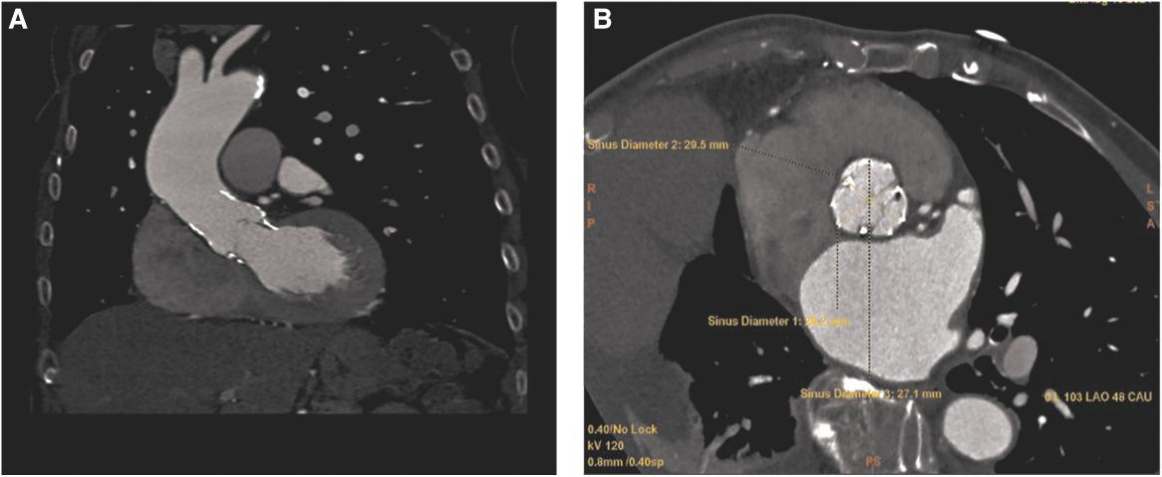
Preprocedural multidetector computed tomography planning. (*A*) Measurement of the intended landing zone corresponding to the inflow ring of the sutureless Perceval bioprosthesis. (*B*) Assessment of aortic root dimensions, including annular geometry, to guide valve sizing for valve-in-valve transcatheter aortic valve implantation.
